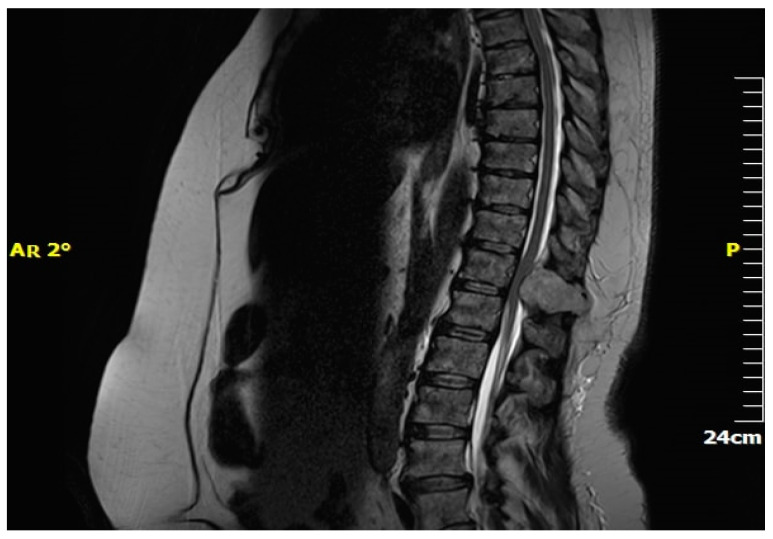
MRI—Spinal cord compression due to metastatic renal tumour.
